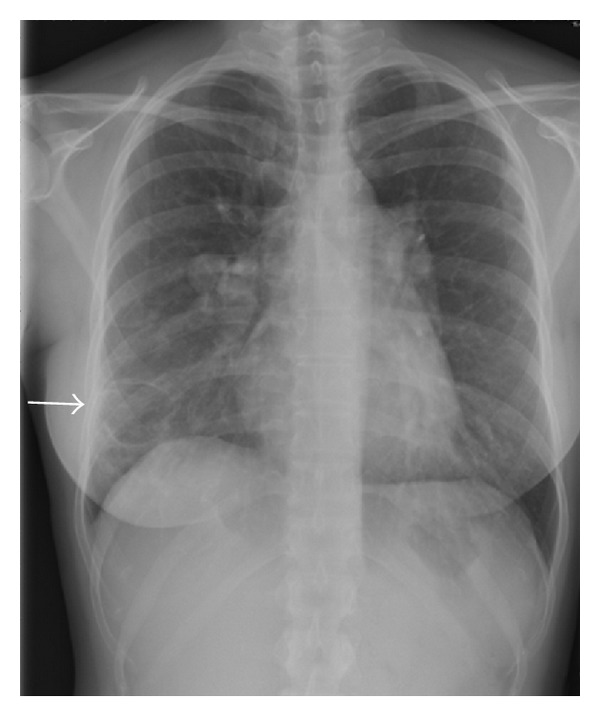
Follow-up chest X-ray shows a thin-walled cavitary lesion in the right lower lobe (white arrow).
